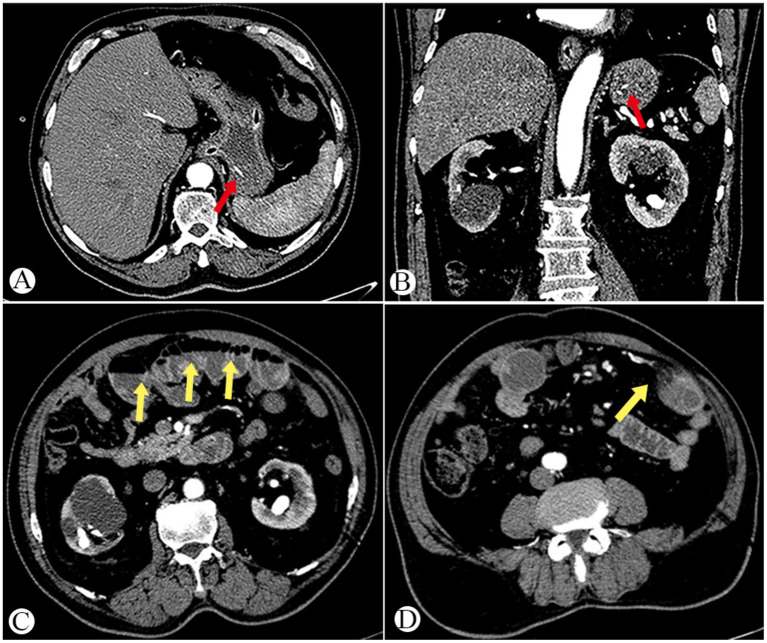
Preoperative abdominal CT findings. (A,B) Strip-like high-density foreign body in the gastric cavity (red arrows). (C) Local gas-fluid accumulation in the small intestine (yellow arrow). (D) Minimal surrounding exudation in the small intestine (yellow arrow).
